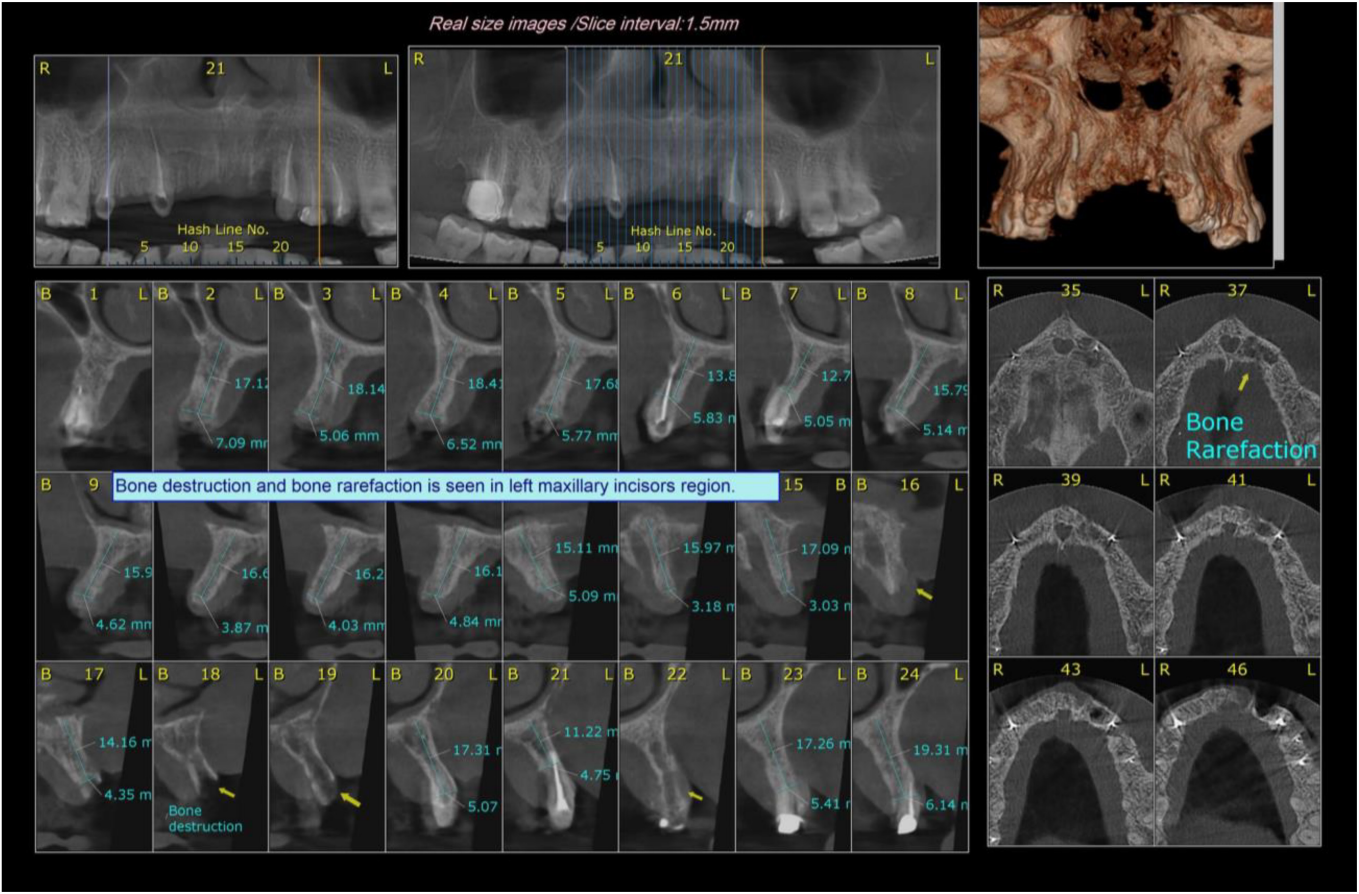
Cone beam computed tomography shows bone destruction in the left maxillary incisor region.
